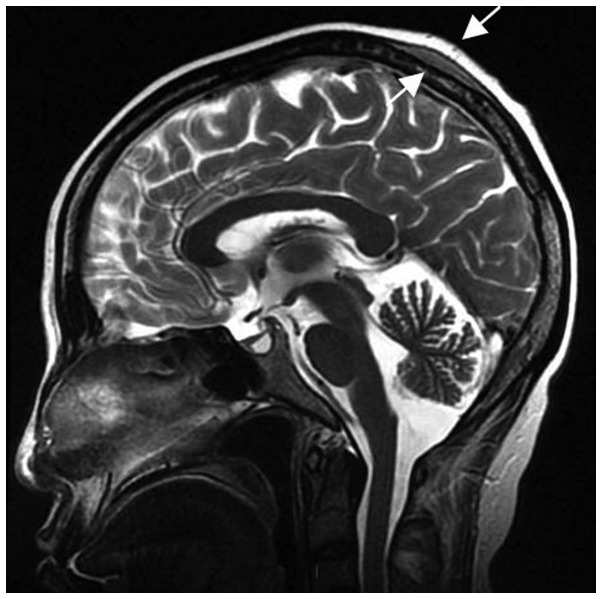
Midline sagittal T2-weighted image captured on December 10, 2010 shows localized thickening of the subparietal galea aponeurotica with homogeneously slightly high signal intensity, as indicated by the white arrow.
